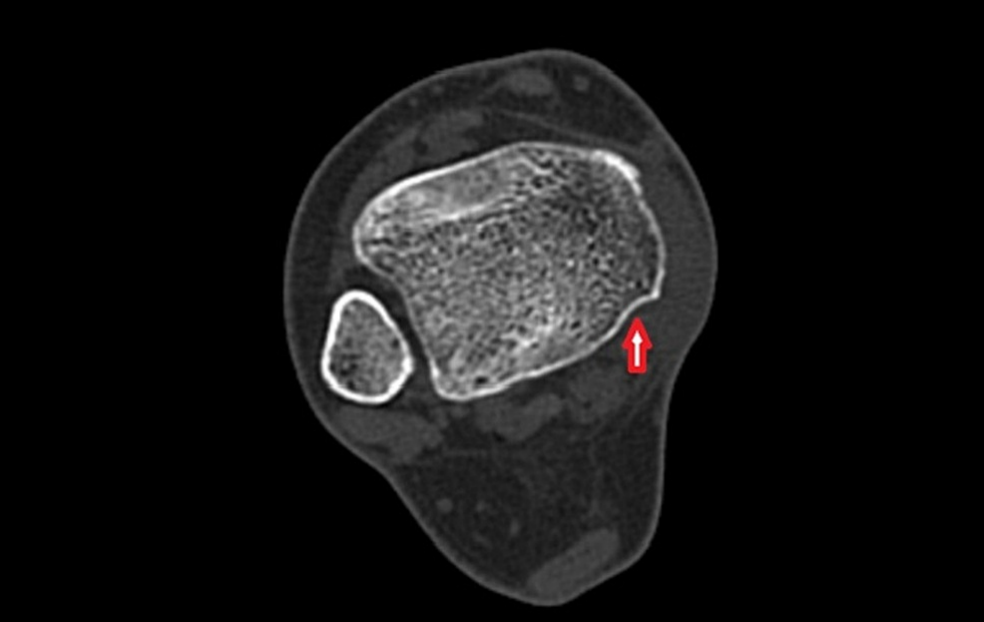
CT scan – axial image showcasing a slightly shallow retromalleolar sulcus (red arrow). CT: Computed Tomography
